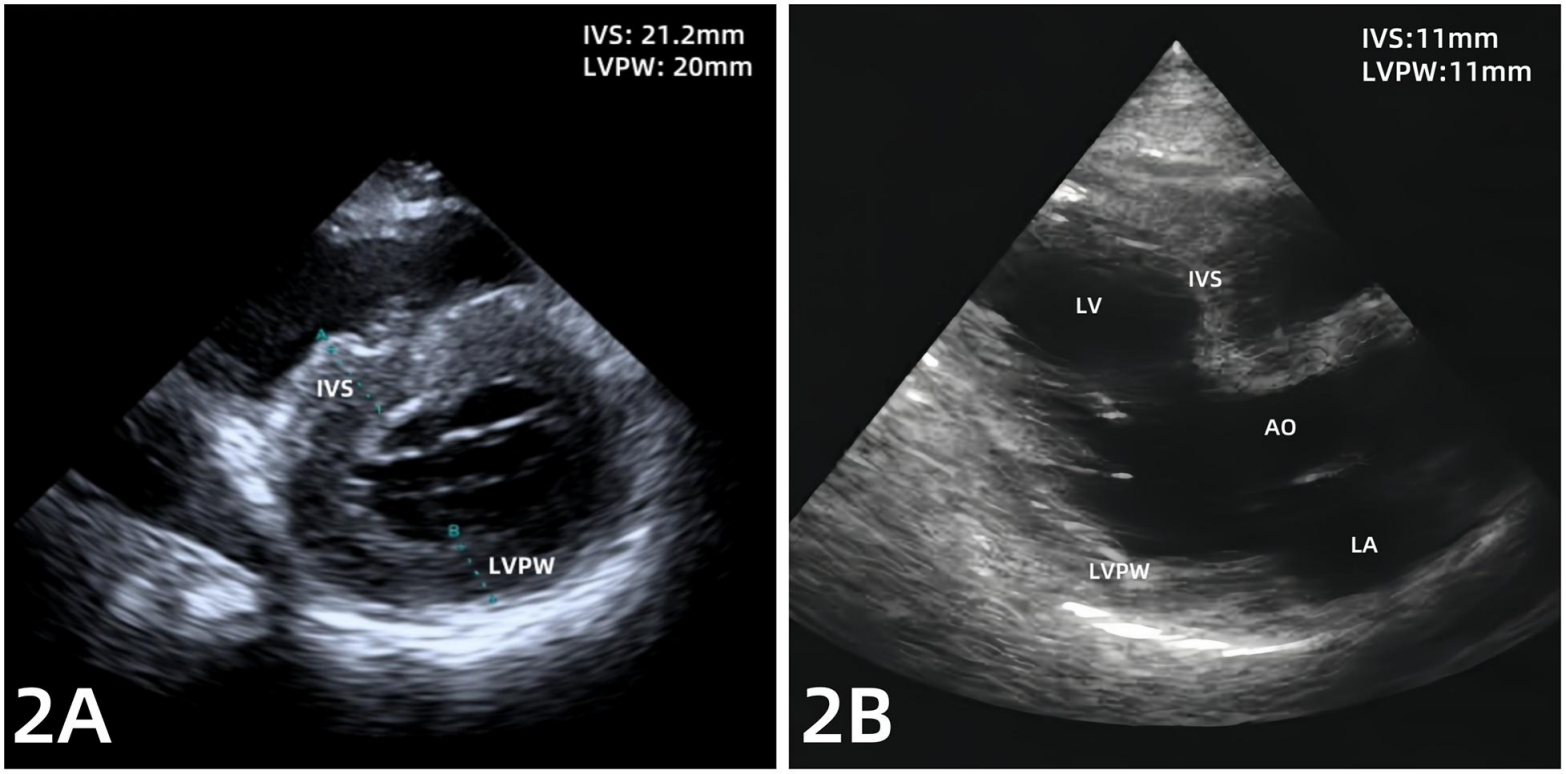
(A) Echocardiography on admission showed interventricular septum (IVS) of 21.2 mm and a left ventricular posterior wall (LVPW) of 20 mm. (B) Non-hypertrophied myocardium on echocardiography 2 years ago.
